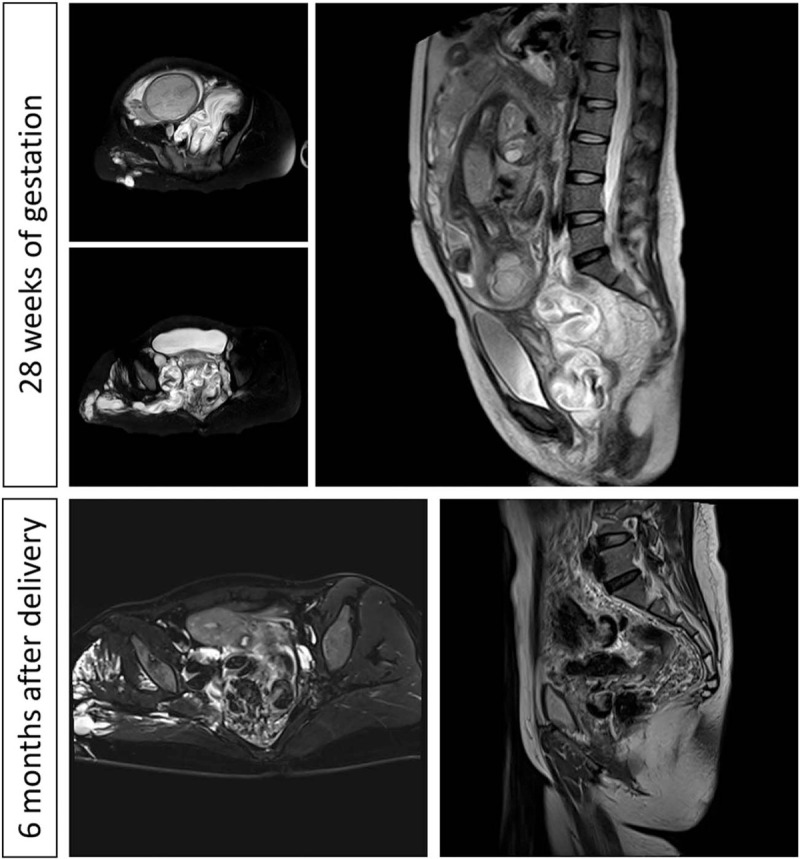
MRI views at 28 weeks of gestation and 6 months after delivery.
